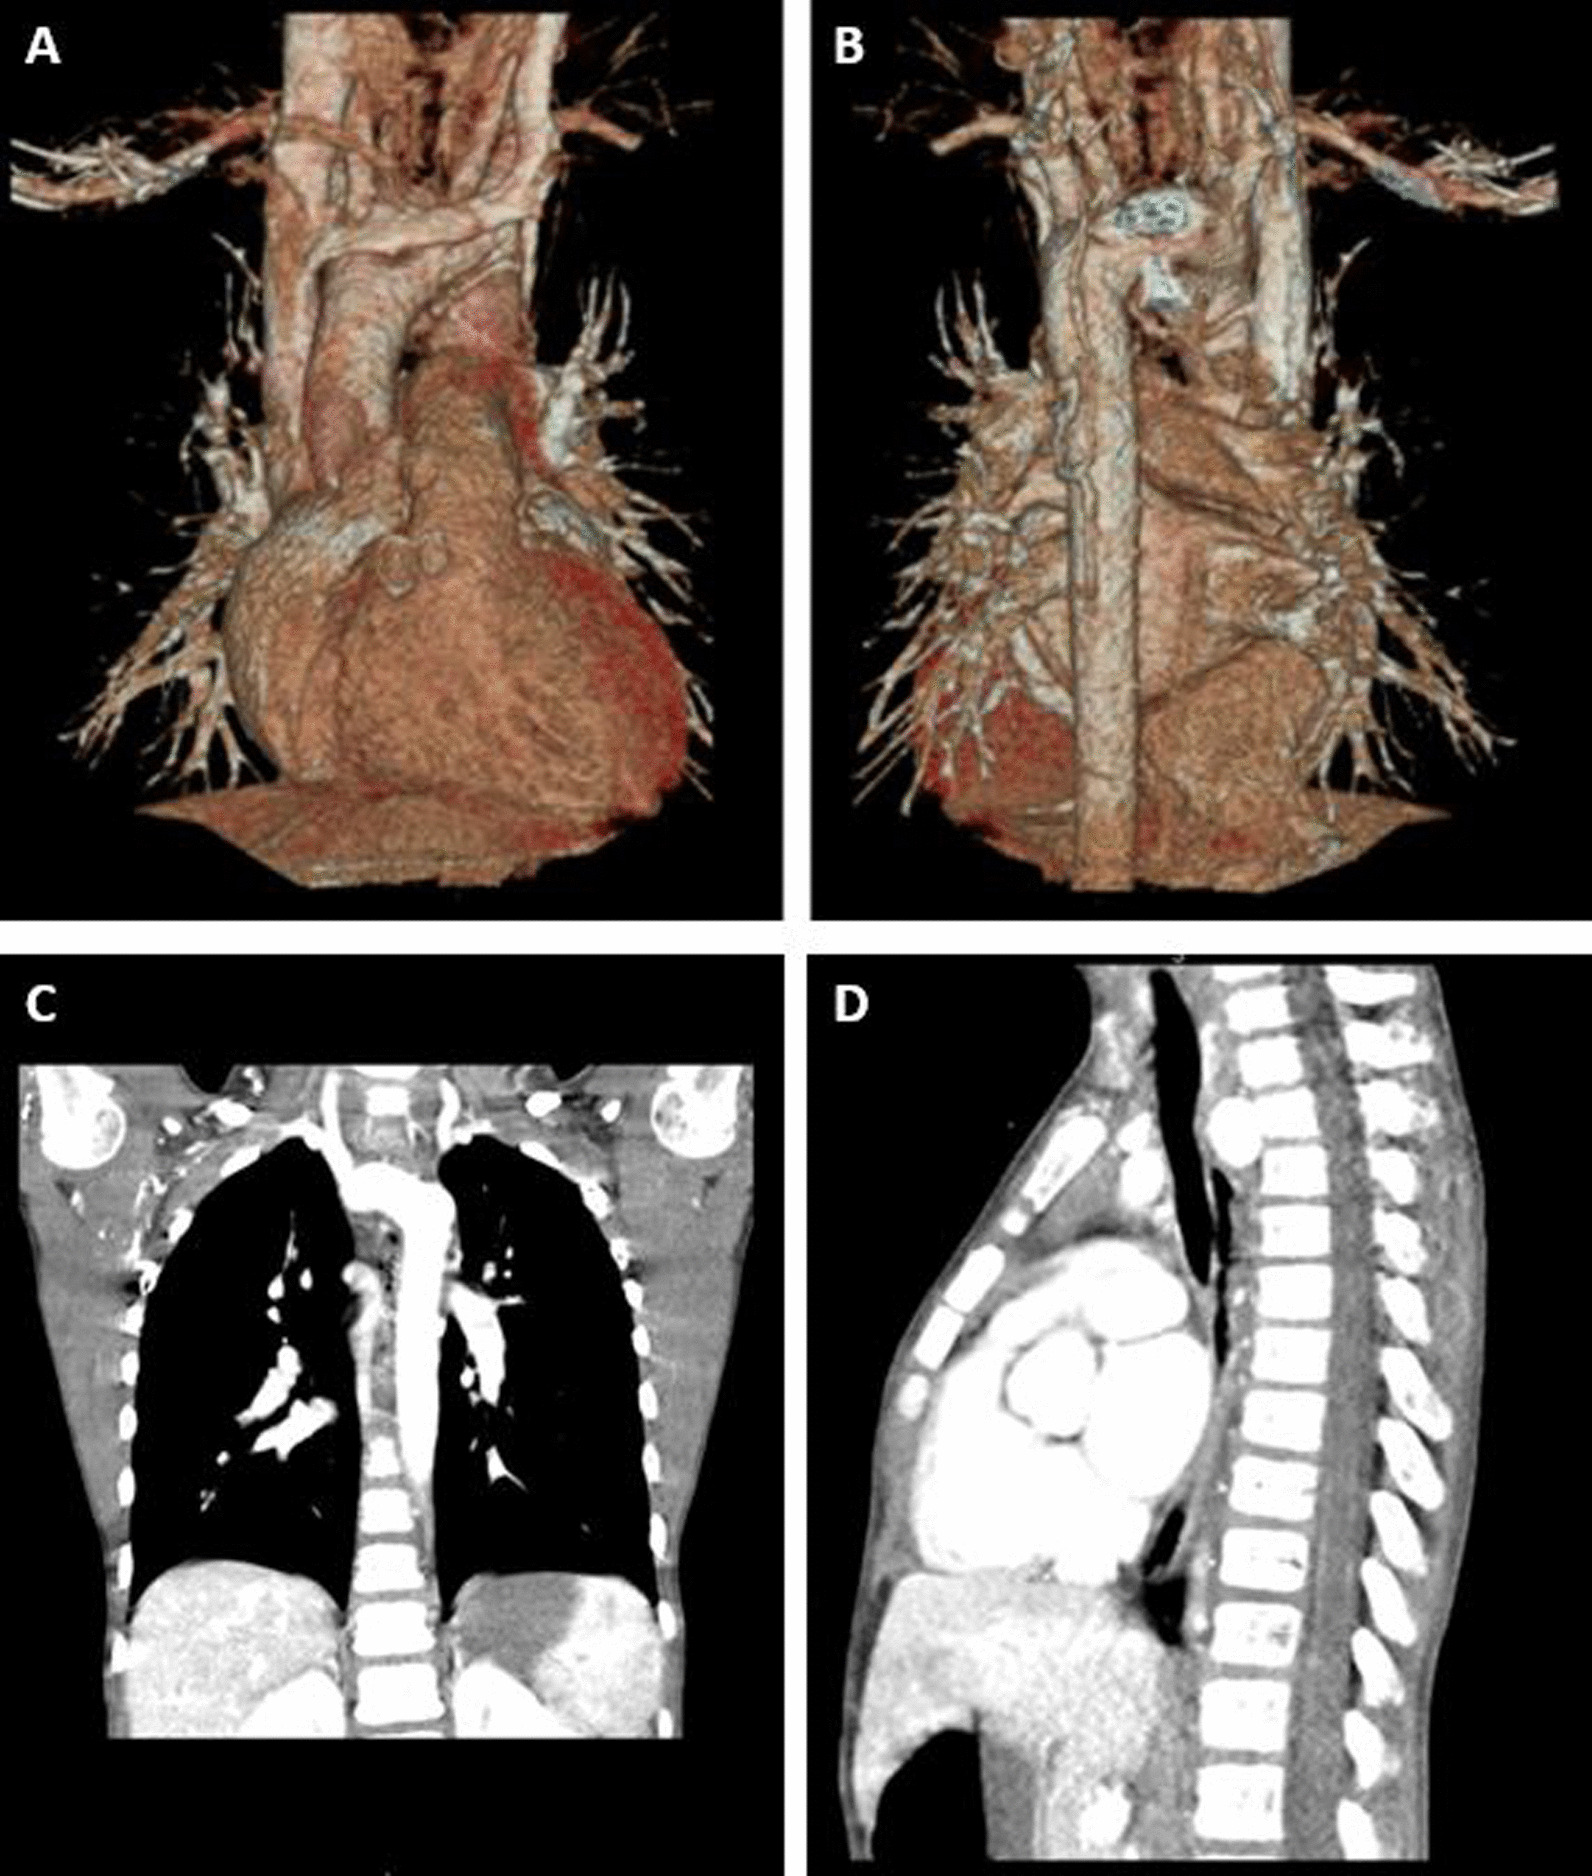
Preoperative imaging. Three-dimensional computed tomographic reconstruction showing A anterior view of double aortic arch, and B posterior view of circumflex aorta. C Coronal and D Sagittal computed tomographic images of circumflex aorta crossing right to left posterior to the trachea and superior to the carina
